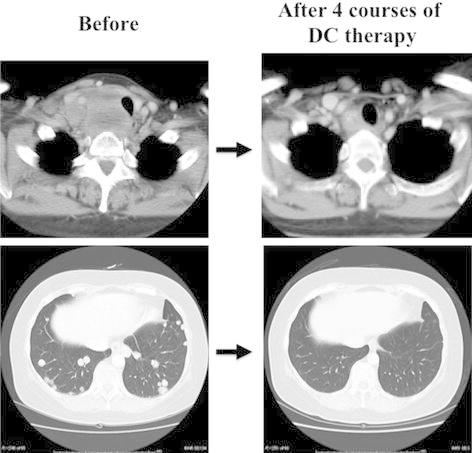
Computed tomography at the thyroid level and lung in a patient who received chemotherapy with docetaxel and cisplatin (DC therapy) as induction therapy (Case 3). The primary thyroid lesion showed a partial response, and the lung metastasis showed a complete response
